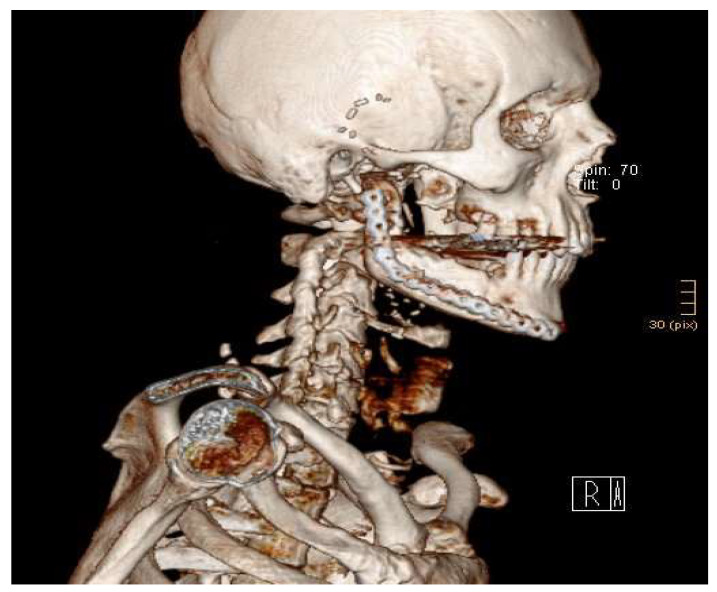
The 3D reconstruction of a CT scan showing an anatomically correct reconstruction of the right mandible up to the condylar head with the sequential chimeric LFC and DCIA flap.
